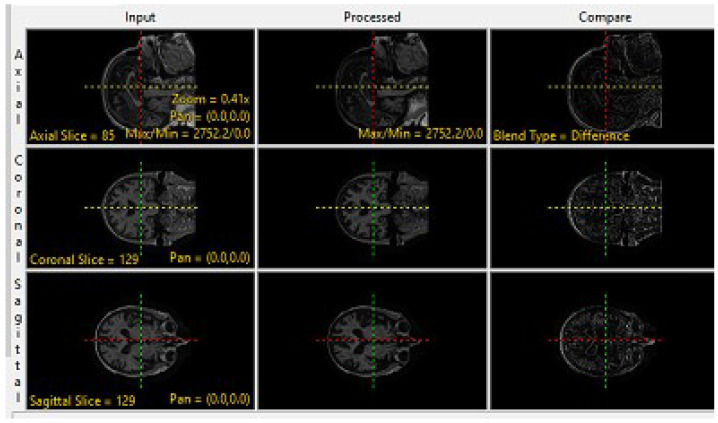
Morphologic Operation for MRI Image.
